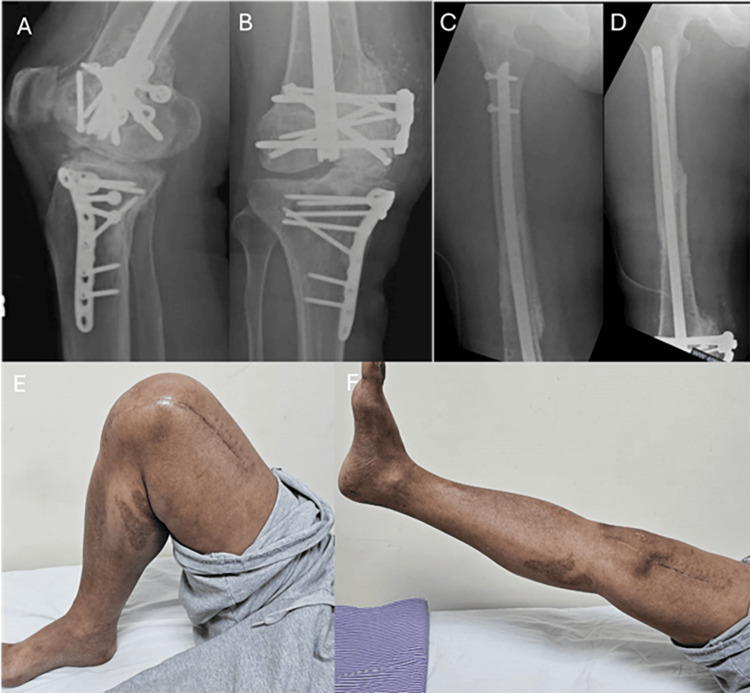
Follow-up radiographs and knee range of motion after four months (A) Plain radiograph of right knee (lateral view) indicating union of Hoffa fracture; (B) Plain radiograph of right knee (AP view) indicating union of Hoffa fracture; (C) Plain radiograph of right femur (lateral view) indicating union of femur fracture; (D) Plain radiograph of right femur (AP view) indicating union of femur fracture; (E) and (F) Clinical photograph demonstrating full right knee range of motion
